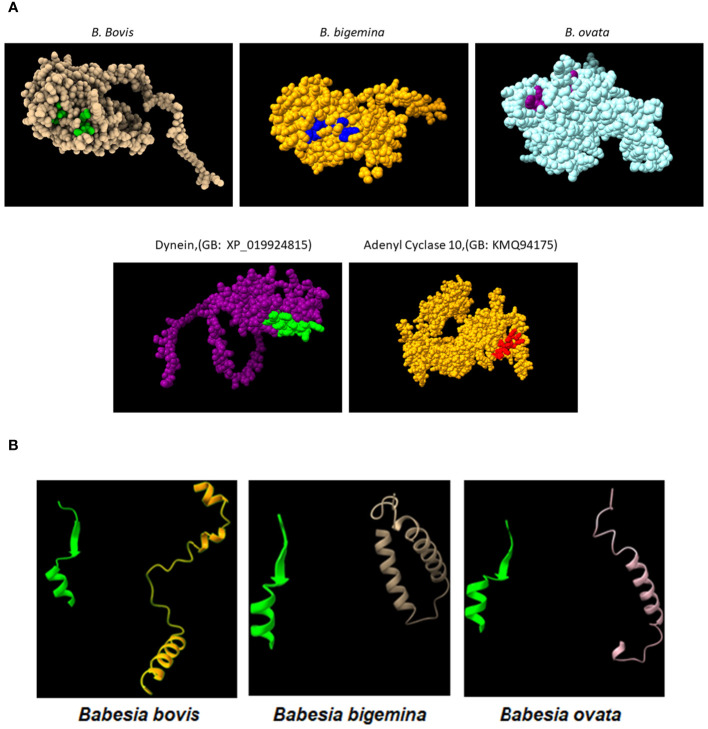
(A) Upper panel: AlphaFold generated surface structure predictions of the RRA derived from (*B*) *bovis*, (*B*) *bigemina*, and (*B*) *ovata*. Residues in green, blue and purple color correspond to surface exposed residues of the 15-mer motif of RRA. Lower panel: AlphaFold generated surface structure predictions of Dynein (GenBank access #: XP_019924815), and Adenyl Cyclase 10 (GenBank access #:KMQ94175). The areas marked in green and red correspond to surface exposed sequences of the conserved 15mer sequences in these two proteins. (B) Conserved Alphafold predicted structural features of the 15-mer motif in the (*B*) *bovis*, (*B*) *bigemina*, and (*B*) *ovata* RRA proteins (green) compared with their structurally distinct CT regions (non-yellow colors).
